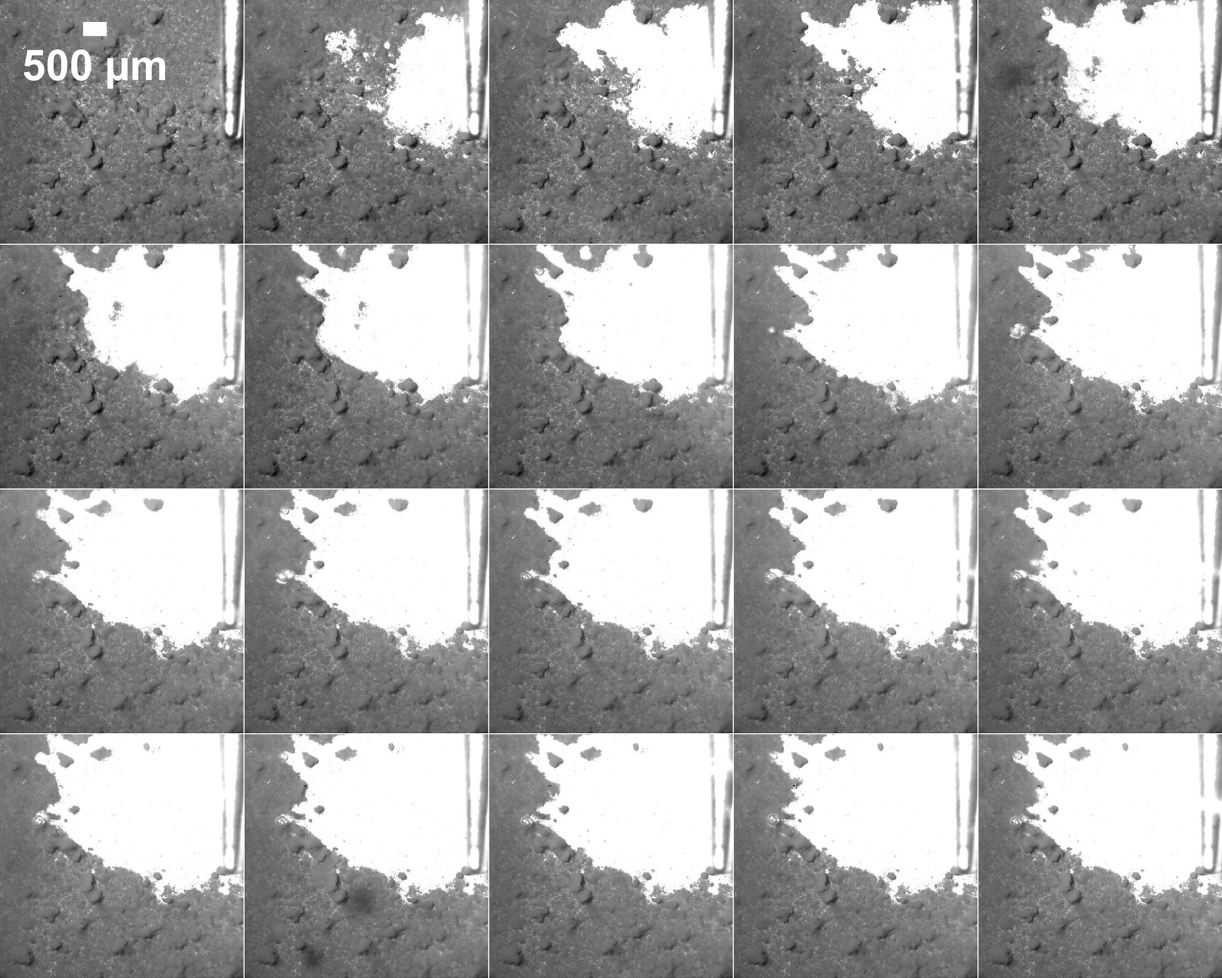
High speed video stills showing biofilm being removed from a rough Ti surface using ultrasonic cavitation at a standoff distance of 0.5 mm. Time between frames is 0.1 s (total 2 s). The cleaned area is white and the dark area is where biofilm is still attached to the surface. See S1 Video for high speed videos of all surfaces at all standoff distances.
